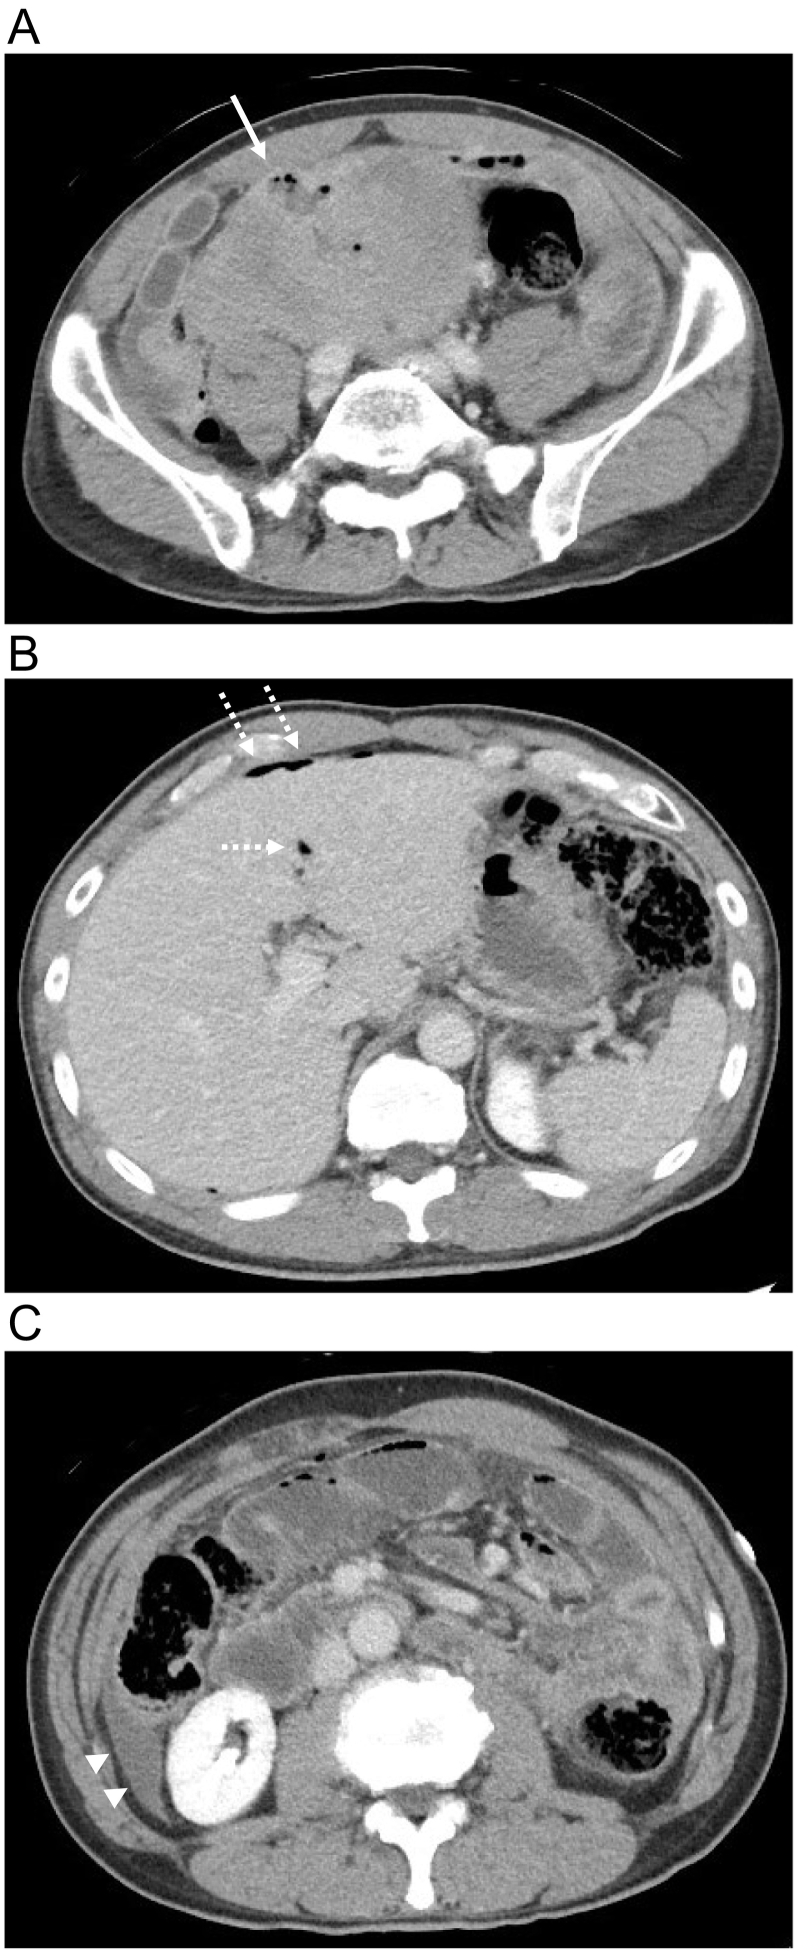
(A) CT revealed a 15 × 15 × 14-cm large internal heterogeneous intra-abdominal tumor with a mosaic-like contrast effect in the pelvis and the lower right abdomen, and abscess formation due to tumor necrosis was present on the ventral surface layer (arrows). Free air (B: dotted arrows) and ascites (C: arrowheads) were found in the abdominal cavity. Although the small intestine appeared enlarged as a whole (C).
